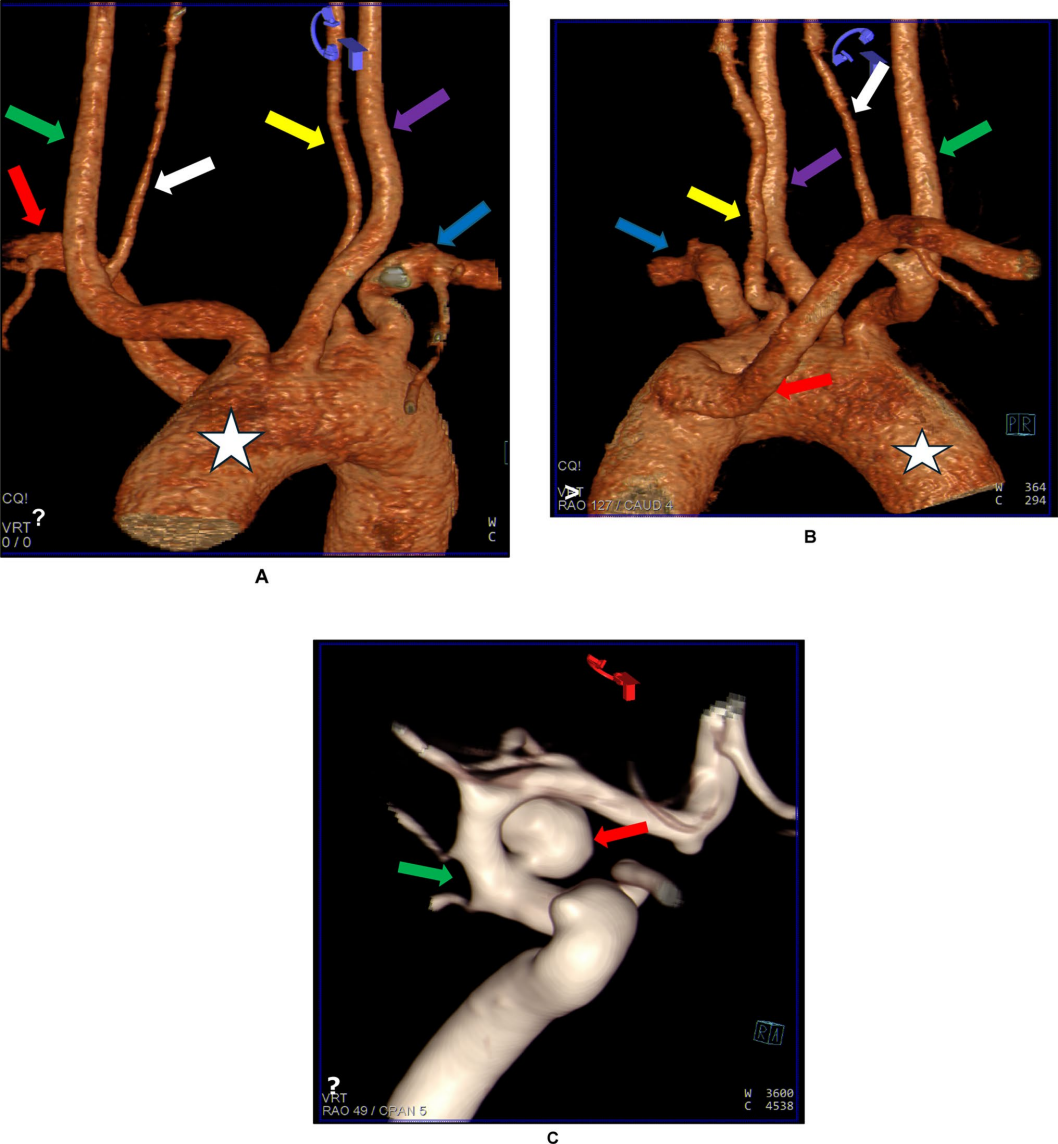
A 3D-CTA reconstructed image of the aortic arch (white star) showing the anterolateral view of the arch of the aorta and its branches. An absent brachiocephalic trunk was noticed. The first branch of the arch of the aorta is the right common carotid artery (RCCA-green arrow). The right vertebral artery arises from the anteromedial part of the RCCA (white arrow). The RCCA was followed by the left common carotid artery (LCCA-purple arrow). The left VA directly originates from the arch of the aorta (yellow arrow). The left subclavian artery (LSCA) is the last branch from the anterior view (blue arrow). An aberrant right subclavian artery (RSCA) (arising from the posterior aspect of the arch of the aorta took its origin proximal to the origin of the left subclavian artery (distal portion of the AA) (red arrow). B 3D-CTA reconstructed image of the aortic arch (white star) showing the posterior view of the arch of the aorta and its branches. An absent brachiocephalic trunk was noticed. The first branch of the arch of the aorta is the right common carotid artery (RCCA-green arrow). The right vertebral artery arises from the anteromedial part of the RCCA (white arrow). The RCCA was followed by the left common carotid artery (LCCA-purple arrow). The left VA directly originates from the arch of the aorta (yellow arrow). The left subclavian artery (LSCA) is the last branch from the anterior view (blue arrow). An aberrant right subclavian artery (RSCA) (arising from the posterior aspect of the AA) took its origin proximal to the origin of the left subclavian artery (distal portion of the AA) (red arrow). C 3D-CTA volume reconstruction of the right ICA run showing the superiorly pointing, posterior communicating artery aneurysm (red arrow) arising from the medial wall of the ICA (green arrow).
